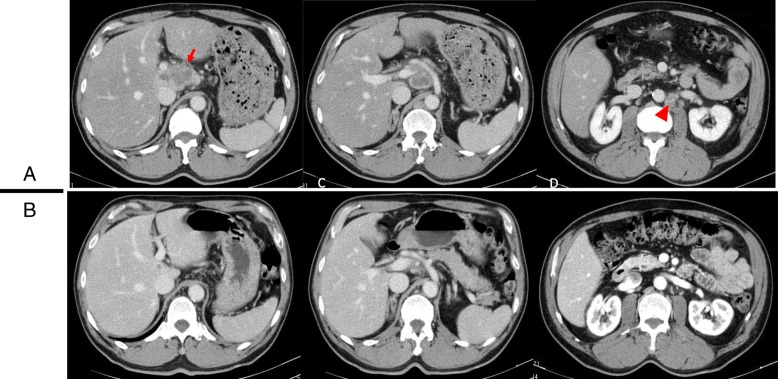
Pre- and post-chemotherapy enhanced computed tomography scan findings. a A hypovascular mass in the segment 1 (arrow), which invaded to the portal vein and showed multiple lymph node metastases including para-aortic lymph node (arrowheads). b Following chemotherapy, the complete response of para-aortic lymph node metastases was achieved
